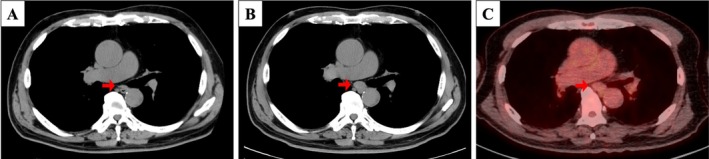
Chest computed tomography (CT) 6 months before showed no mediastinal abnormalities (A). On admission, chest CT revealed subcarinal (station 7) lymphadenopathy (B). ^18^F‐fluorodeoxyglucose positron emission tomography/computed tomography (^18^F‐FDG PET/CT) demonstrated no abnormal ^18^F‐FDG uptake in the station 7 lymph node (C). The station 7 lymph node is indicated by an arrow.
